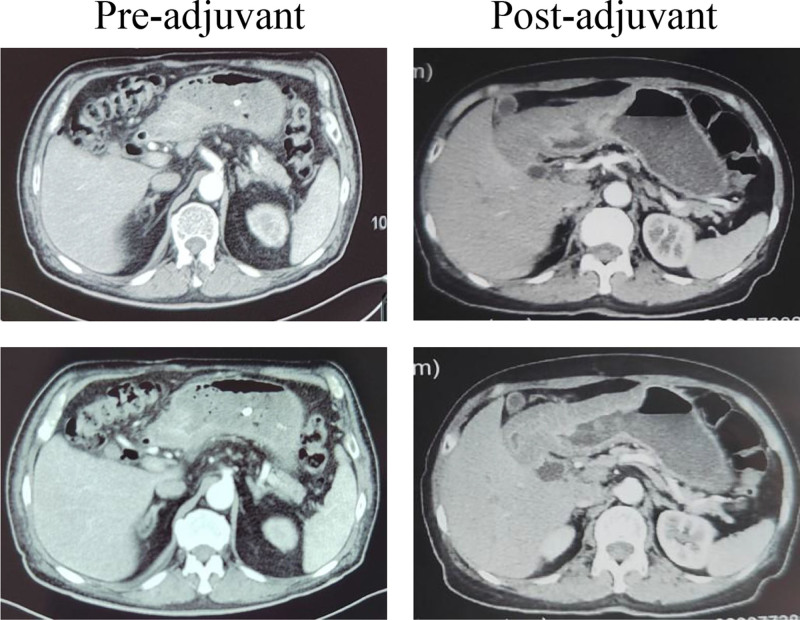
CT scan of the patients who achieved SD after neoadjuvant treatment and the patient classified with a TRG2 pathological response. CT = computed tomography, SD = stable disease, TRG2 = tumor regression grading 2.
